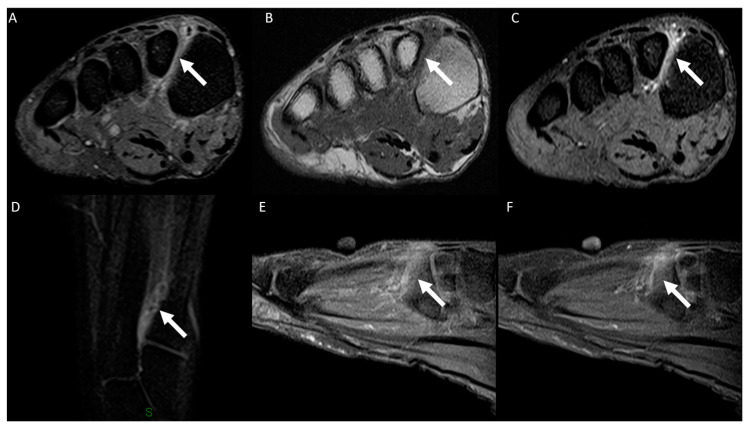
A 54-year-old woman presented with pain in her right foot while walking, persisting for 15 days. Forefoot MRI images in the short-axis plane, including a T2-weighted fat-suppressed scan (A) and a T1-weighted scan (B), as well as a post-gadolinium T1-weighted fat-suppressed scan (C), and in the long-axis plane, a post-gadolinium T1-weighted fat-suppressed scan (D), along with a sagittal T2-weighted fat-suppressed scan (E) and a post-gadolinium T1-weighted fat-suppressed scan (F), revealed thrombosis in the perforating vein of the first space (indicated by arrows). This condition was characterized by venous dilation, with intraluminal thrombus and an intravenous filling defect.
